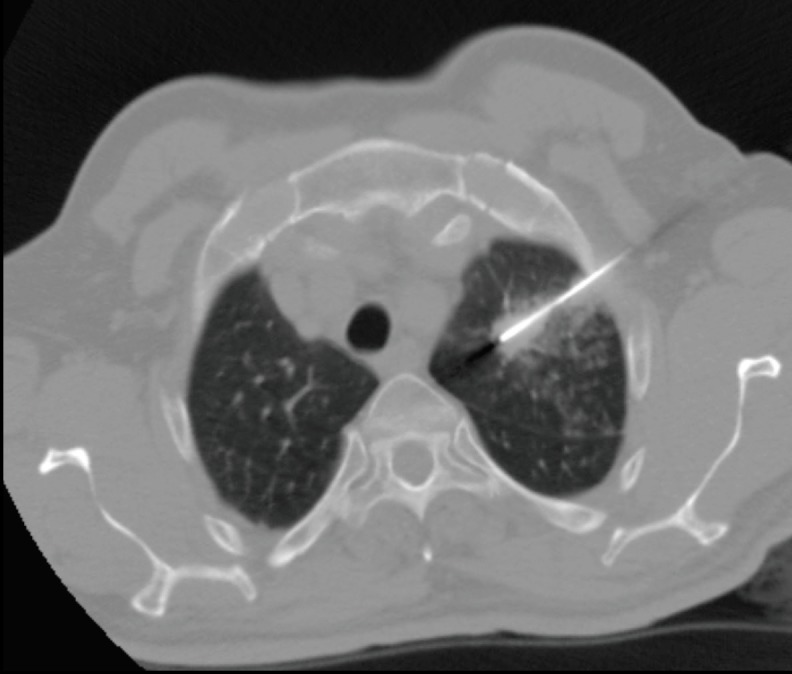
CT-guided lung biopsy of left sided 16 mm pulmonary
